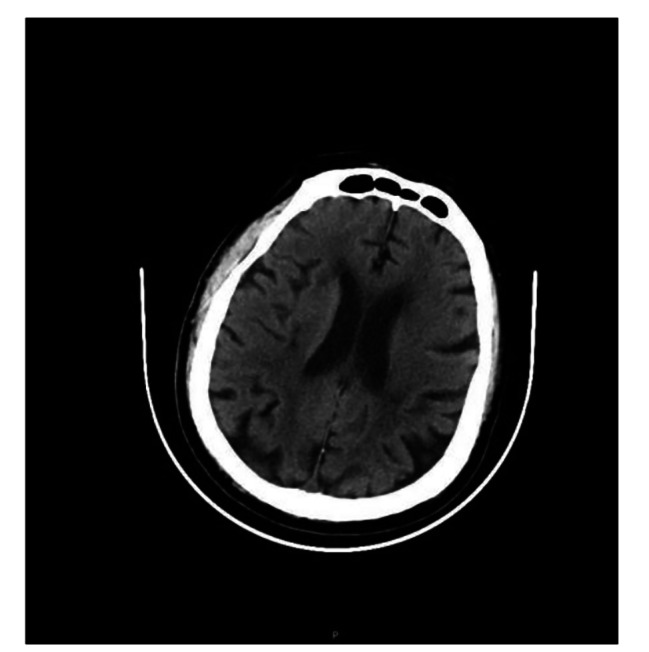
The patient’s brain CT scan on ICU admission
